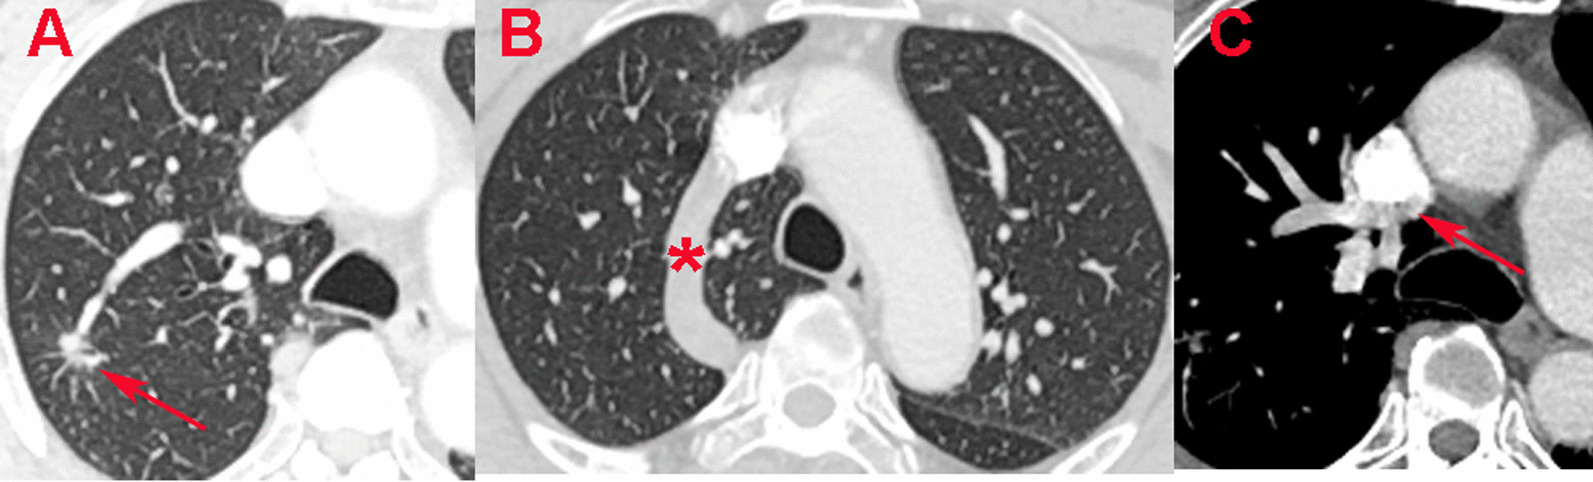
a The malignant nodule in in two-dimensional CT scans; b asterisk represents the dilated azygos vein; c The red arrow represents partial anomalous pulmonary venous return (PAPVR) drainages into superior vena cava
